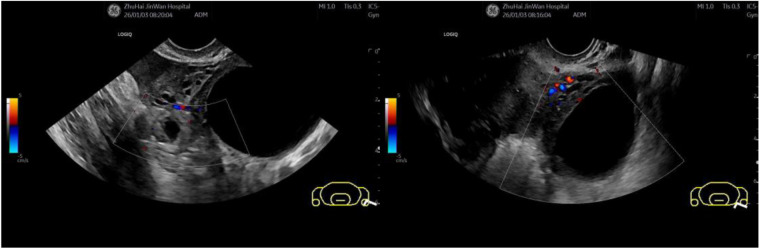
Pre-treatment transvaginal ultrasound (January 3, 2026). A 21 × 15 mm mixed echogenic mass adjacent to the left ovary contains a 10 × 6 mm gestational sac with a yolk sac and peripheral blood flow signals.
